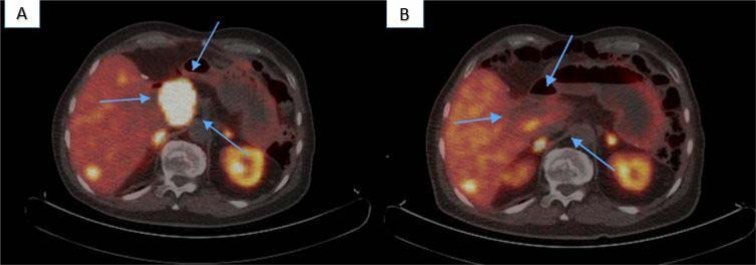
^68^gallium DOTATOC PET/CT; (A): Seven weeks prior to the therapy, revealing intense tracer uptake in the liver lesion (SUV max: 60.78), (B): Twelve months after last PRRT cycle with significant decreased tracer uptake (SUV max: 8.62)
